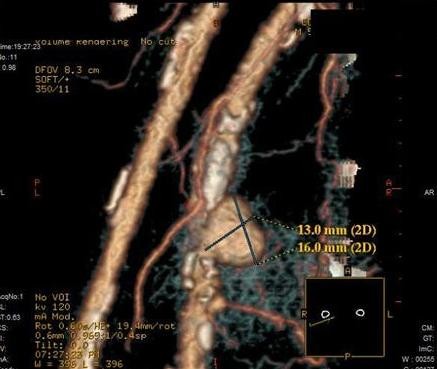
Mycotic aneurysm. CT scan with tridimensional reconstruction showing an aneurysmatic sacular formation in the dependence of the right superficial femoral artery.
